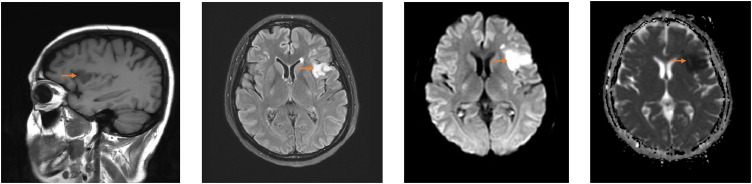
MRI brain from the left: T1-weighted image, T2 FLAIR weighted image, Diffusion-weighted image, and apparent diffusion coefficient map, respectively. Redemonstrations of the acute infarction in the left anterior insular cortex, low signal intensity in T1, high signal intensity on T2, and showing restrictive diffusion.
